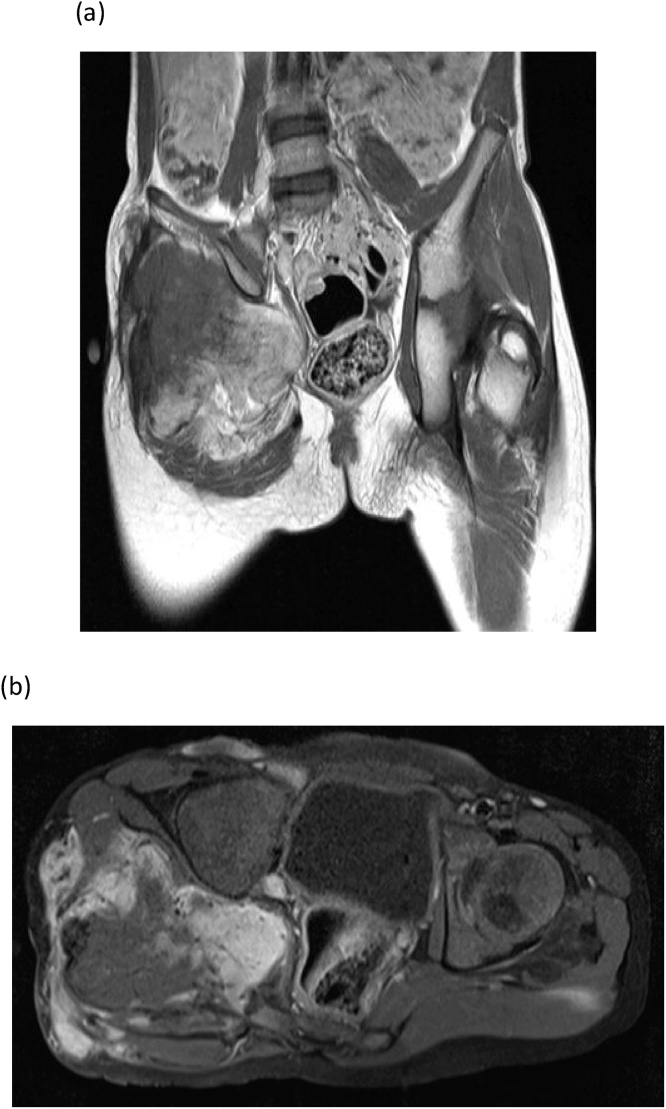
MRI pelvis of fibromatosis of right gluteal region. Coronal plane showing mass infiltrating in the adjacent gluteus muscles and entering in pelvis. Axial plane showing no lymphadenopathy and normal vessels.
